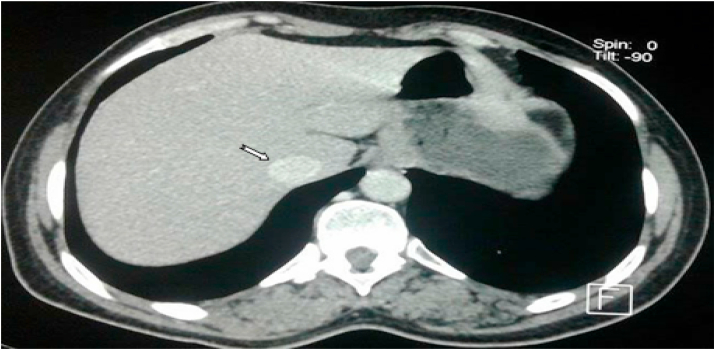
Abdomino-pelvic CT showed well-limited hepatic segment VI damage, discreetly enhancing after injection of PDC.
